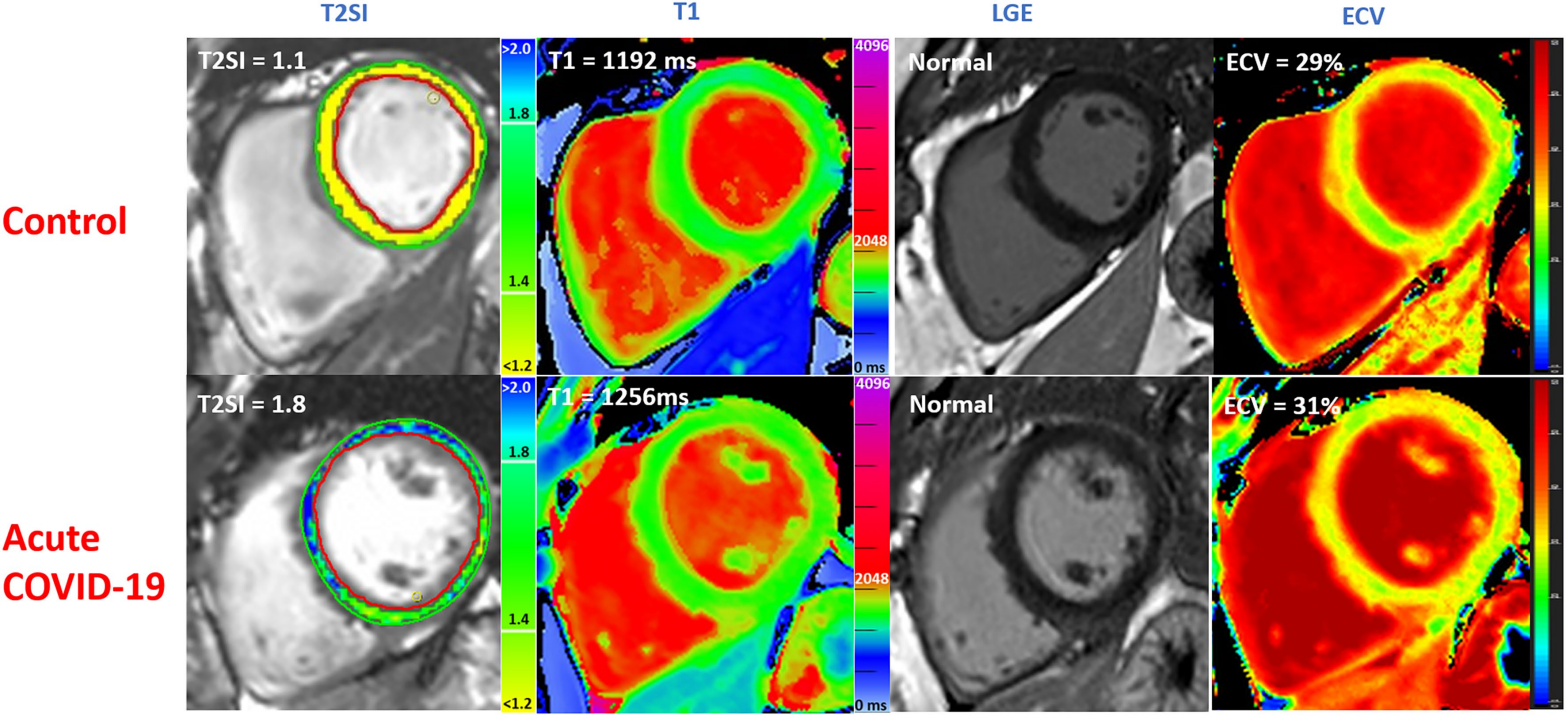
Multiparametric CMR imaging in 3 T MRI scanner comparing acute COVID-19 patients during their hospitalization with matched controls without COVID-19 from the community. T2SI = T2 signal intensity ratio (myocardium: skeletal muscle). LGE, late gadolinium enhancement; ECV, extra cellular volume fraction. Normal values: T1 ≤ 1,244 ms, T2 ≤ 1.4. All values correspond to single slice have been shown.
